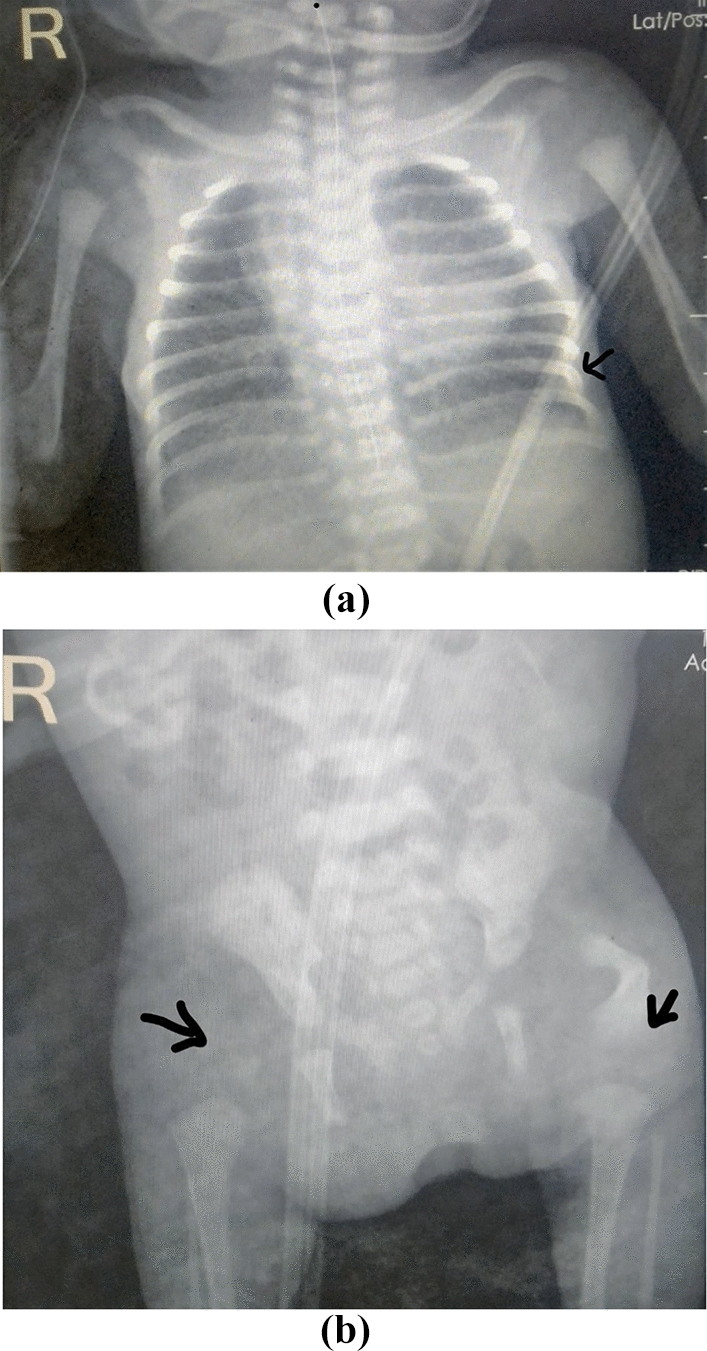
a Anterior–posterior view chest X-ray showing rib deformities (arrow). b Skeletal X-ray showing right femoral aplasia and left femoral hypoplasia (arrows)
